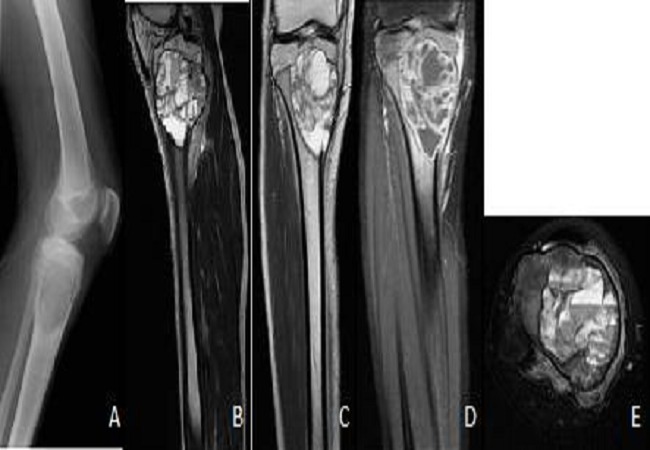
Radiography (A) showing an osteolytic lesion oval metaphyseal tibial very limited. Cuts MRI sagittal T1-weighted (B), coronal T2 (C) and T1 post contrast (D) and axial T2 FATSAT (E) showing the partitions after intralesional contrast enhanced liquid level typical of an aneurysmal cyst
